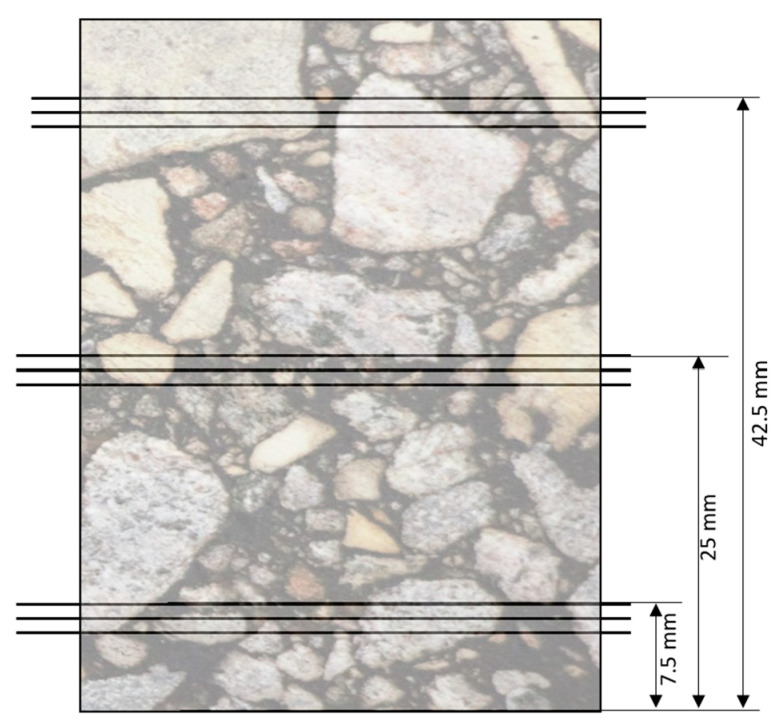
Locations of the selected slices from X-ray CT scans.
